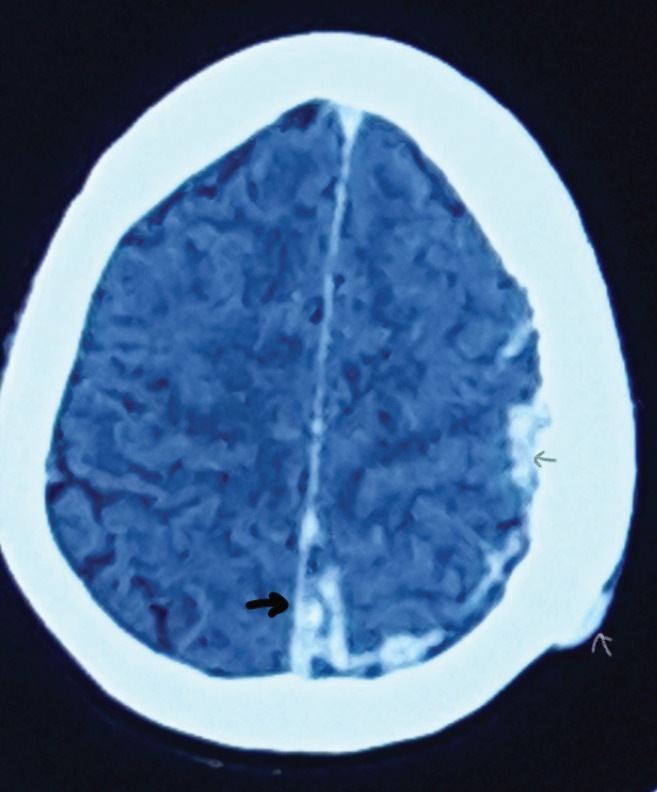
Plain CT scan brain axial view showing similar lesions in tentorium, falx (thick black arrow), dural surface (thin black arrow) and scalp (white arrow) of same side.
